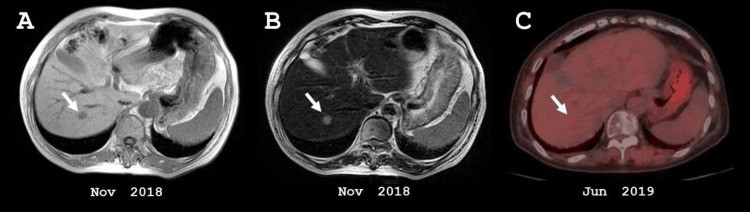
Abdominal MRI and PET-CT before RFA. (A) T1-weighted MRI. (B) T2-weighted MRI. (C) PET-CT showing 18F-FDG uptake (SUVmax = 2.54) in the hepatic metastasis in segment 7 (white arrow). MRI: magnetic resonance imaging, PET: positron emission tomography, SUV: standardized uptake value, FDG: fluorodeoxyglucose.
